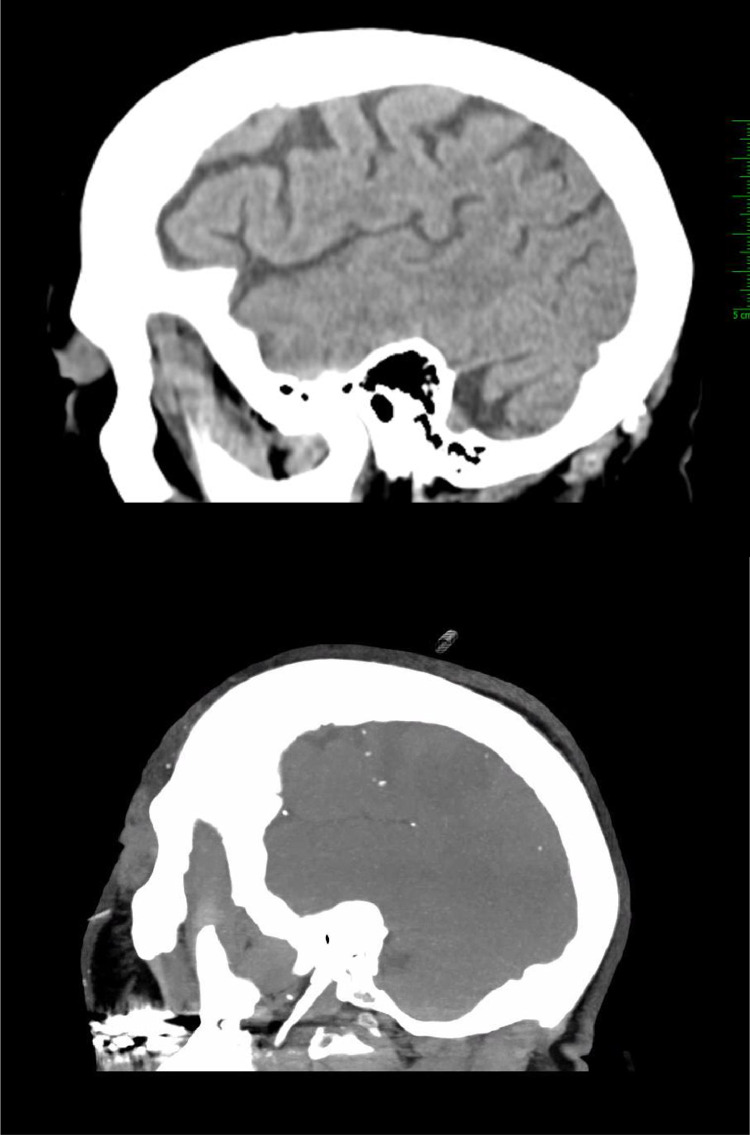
Initial unremarkable sagittal CT head without contrast (left); sagittal MIP reconstruction of follow up CT head without contrast performed 13 h later showing numerous, new calcifications on a single MIP image (right).
